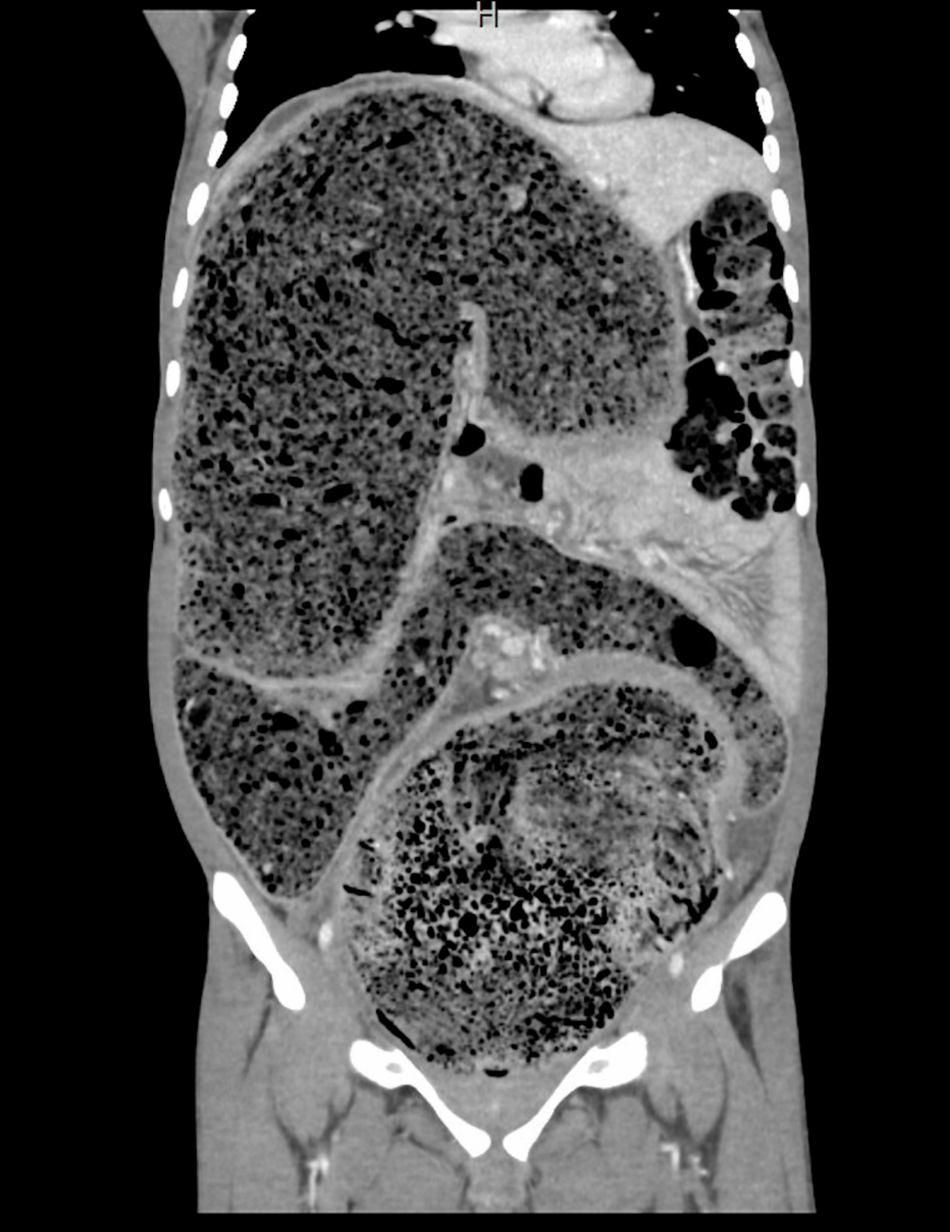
Coronal CT scan with contrast displaying colon fully loaded with feces. The colon is 15-18 cm in width and 60-80 cm in length. This is an image of a huge dilated colon that is loaded with feces.
